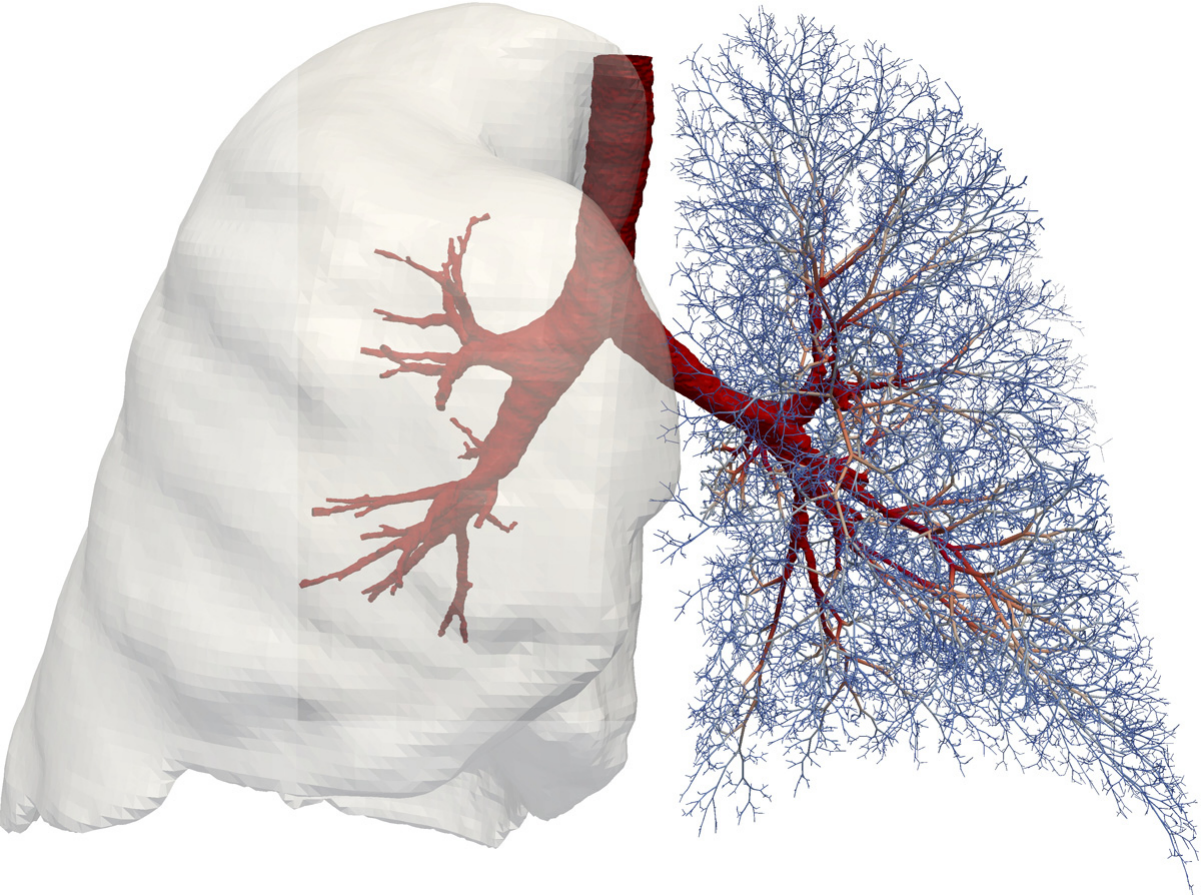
Example of a patient-specific model of an AirPROM subject showing central airways (red) and lung surface (gray) segmented from CT and on the right the 1D volume-filling branching conducting airway network (Figure courtesy of Rafel Bordas, Oxford, www.airprom.eu).
